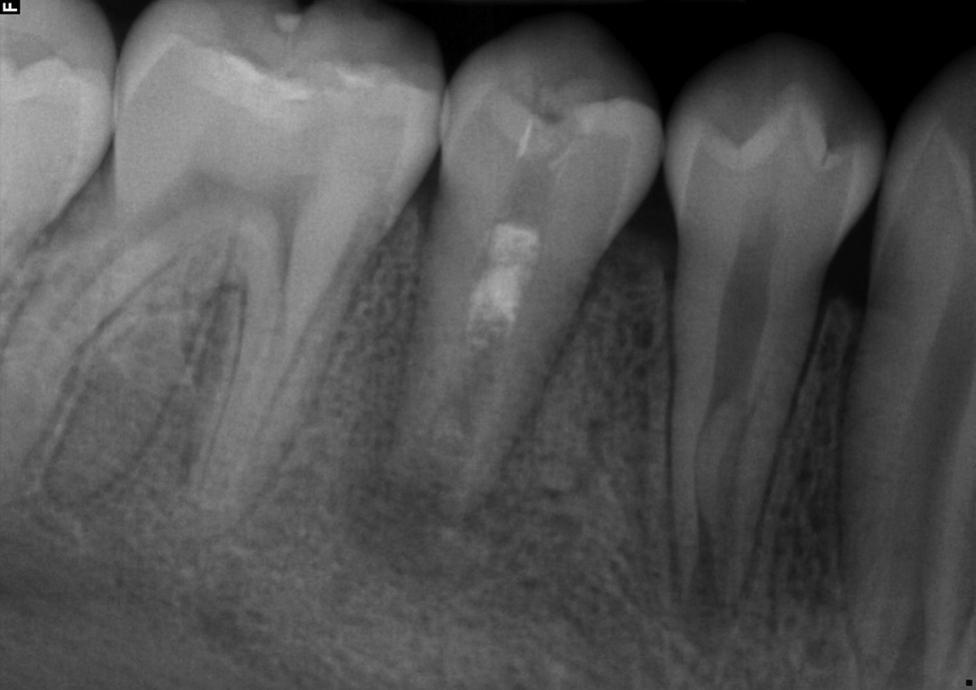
Urgent care periapical film of partially washed-out calcium hydroxide medicament.
